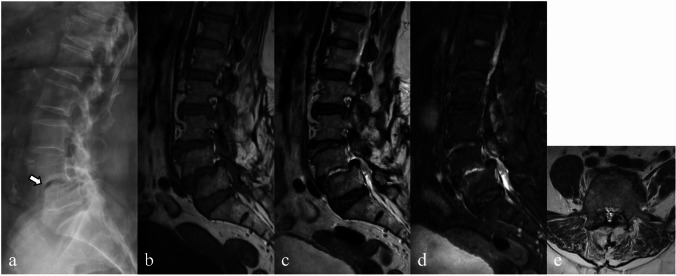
Magnetic resonance images of a 72-year-old female patient with recurrent back pain and a history of decompression surgery performed 20 years ago. Conventional radiograph (lateral view) (a) showing lumbar spondylosis and L4–L5 spondylolisthesis with L4–L5 intradiscal vacuum phenomenon (arrow). Sagittal T1-weighted (b), T2-weighted (c), and short tau inversion recovery (STIR) (d) images show L4–L5 spondylolisthesis with hyperintense T2-weighted/STIR signals in the degenerative disc resembling infectious spondylitis. A relatively preserved dark line of cortical endplate is detected on both T1-weighted and T2-weighted images. An axial T2-weighted image (e) also reveals no abnormal paravertebral or epidural soft tissue to suggest infectious spondylitis. No infection was evident on clinical and follow-up imaging after 2 years
